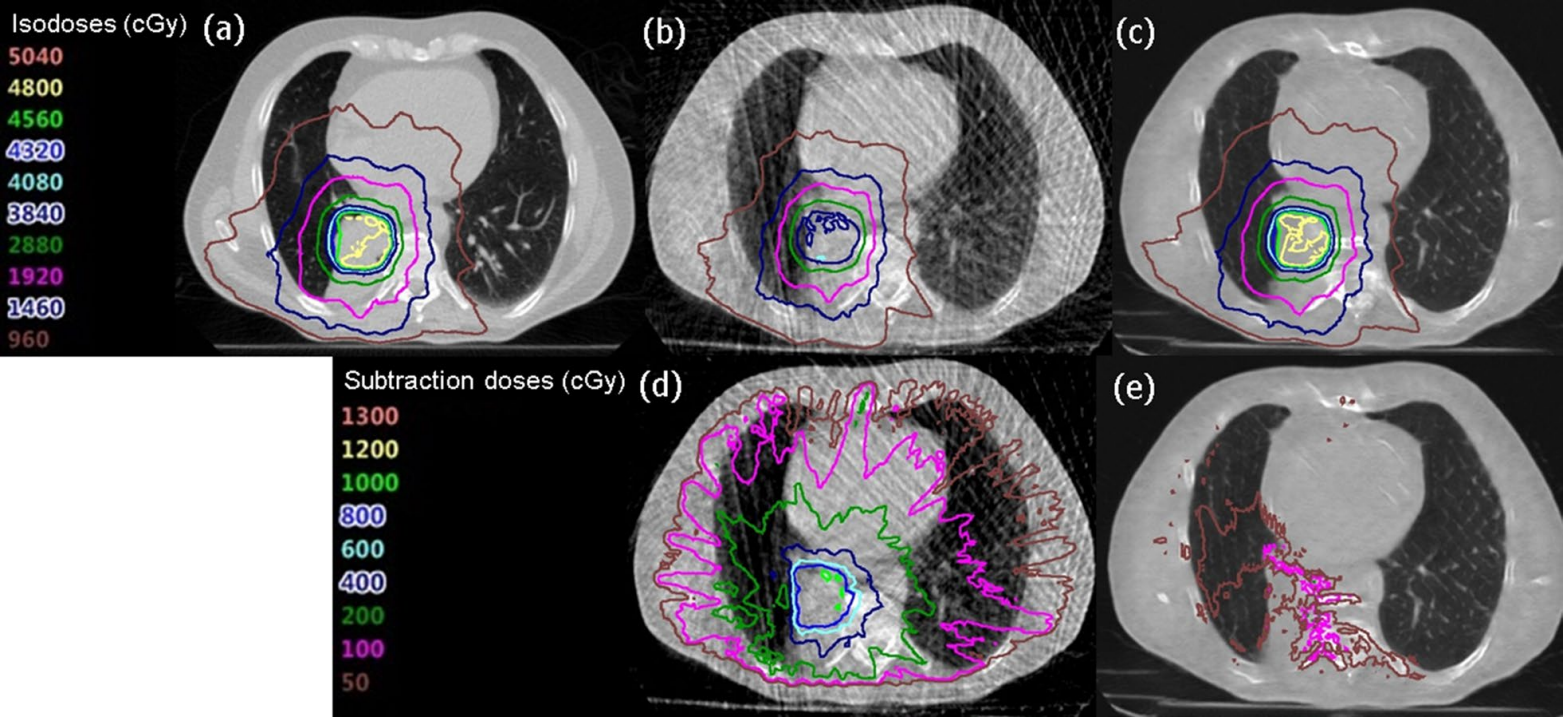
Dose distributions in a volumetric modulation arc therapy (VMAT) plan based on a 4D-MSCT, b 4D-CBCT and c sCT images. Moreover, the dose difference image from the dose distribution of 4D-MSCT image, d 4D-MSCT minus 4D-CBCT, e 4D-MSCT minus sCT
